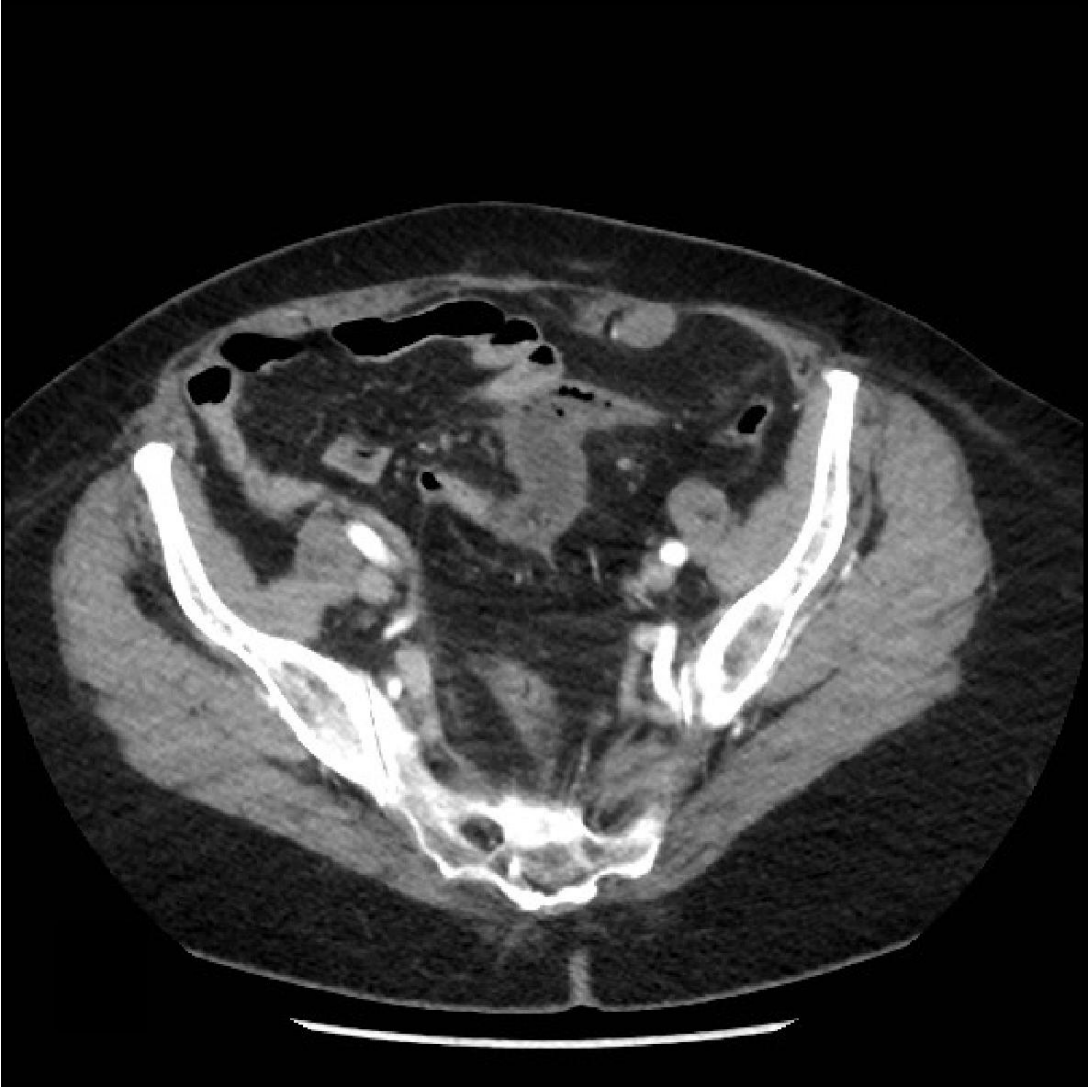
CT of the abdomen after an injection of iodinated contrast material, presenting absent enhancement of the small bowel wall over a segment.
